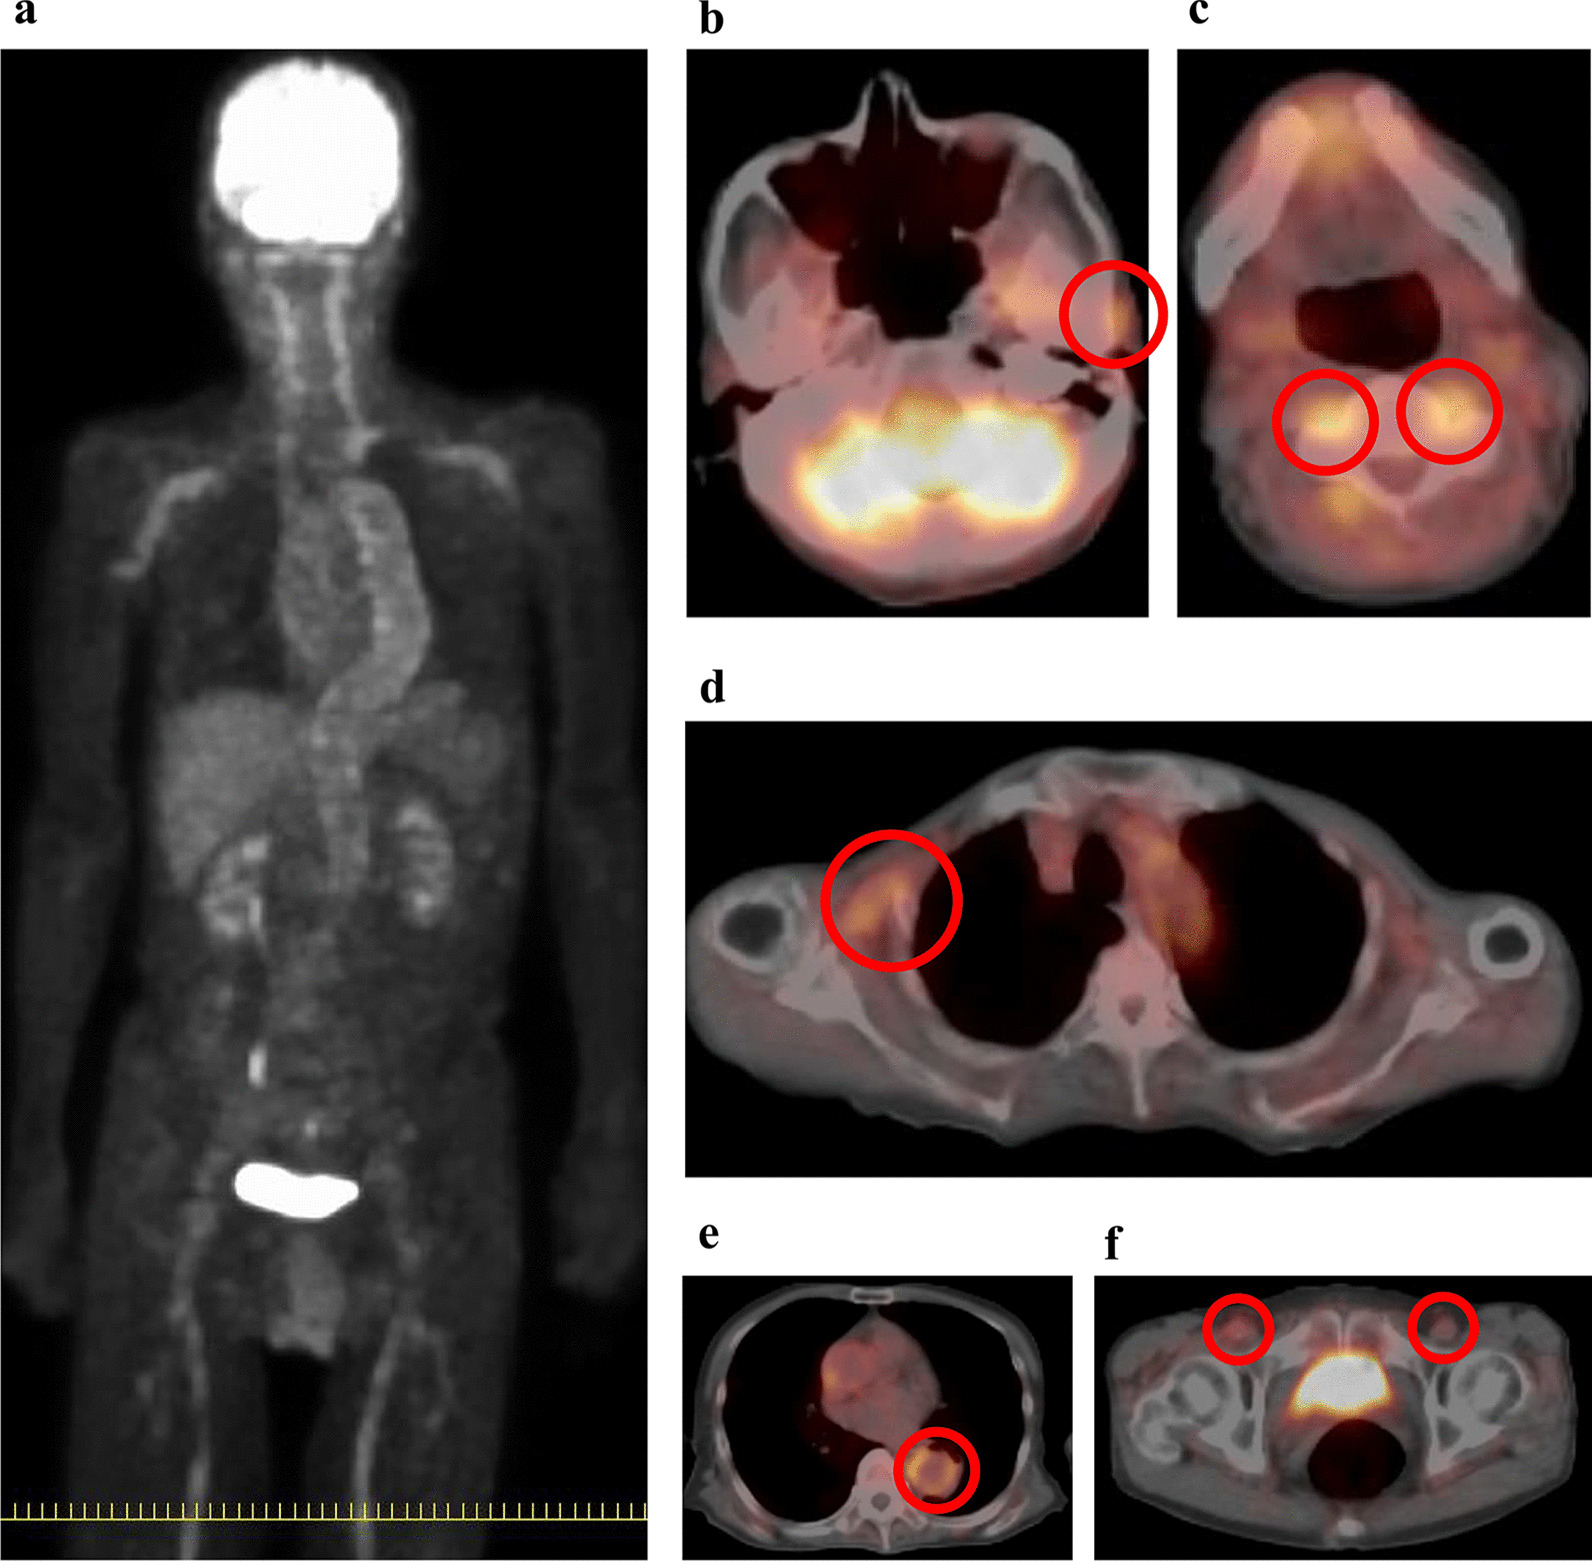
Fluorodeoxyglucose positron emission tomography/computed tomography. a Coronal PET image. b–f Axial PET/CT images. FDG uptake of left temporal artery (b circle, SUVmax 3.6), bilateral vertebral arteries (c circle, SUVmax 4.4), right axillary artery (d circle, SUVmax 3.1), descending aorta (e circle, SUVmax 3.8), and bilateral femoral arteries (f circle, SUVmax 2.3). PET, positron emission tomography; CT, computed tomography; FDG, fluorodeoxyglucose; SUVmax, maximum standardized uptake value
